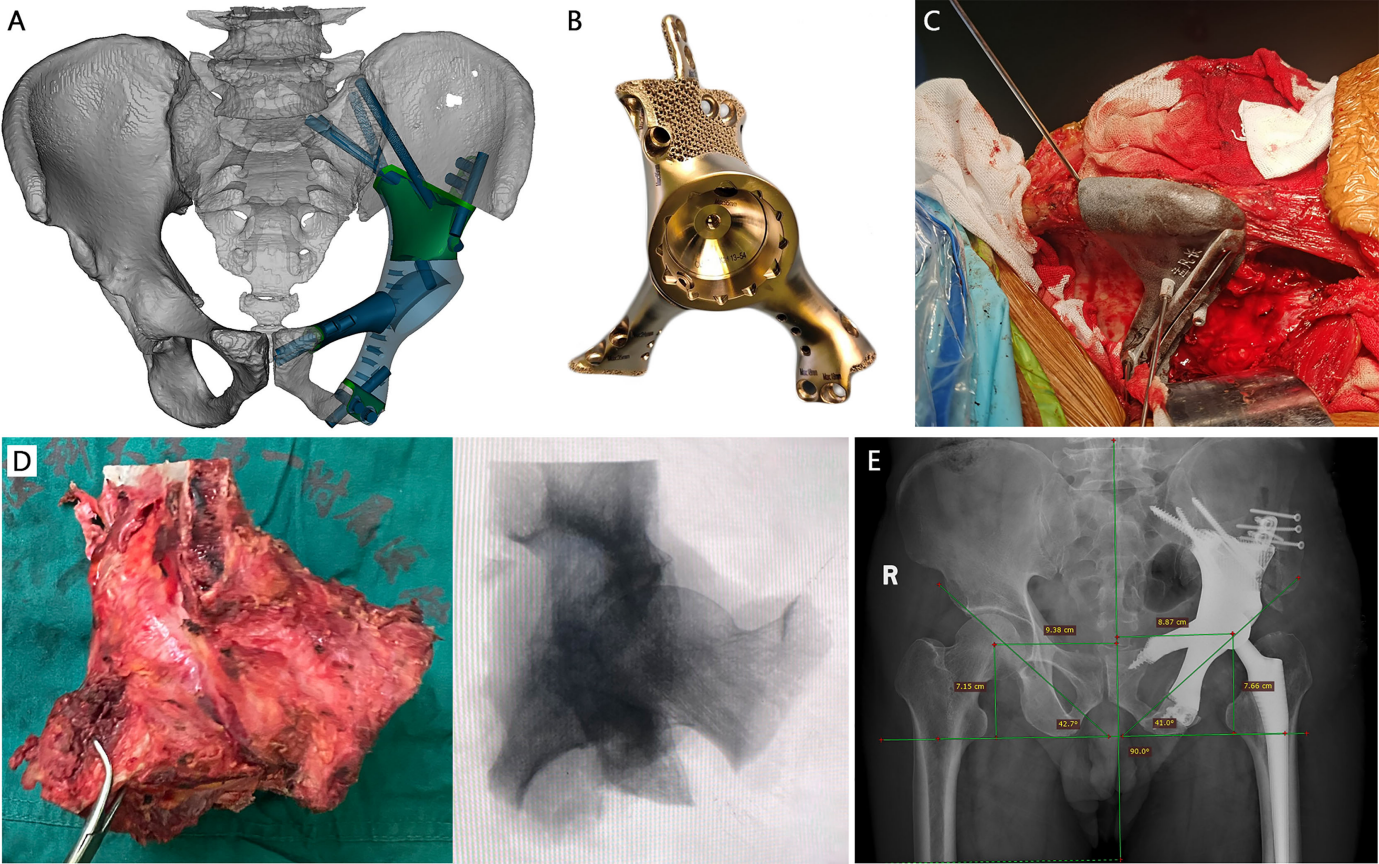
A 66-year-old male patient with metastatic prostate cancer. (A) The design of type B prosthesis after partial type I + II + III resection (green part: porous structure; blue part: solid structure). (B) The real picture of the implant. (C) Intraoperative installation of patient-specific osteotomy guide on the iliac crest. (D) Extra-articular resection of the tumor. (E) Postoperative x-ray manifested promising accuracy of the prosthesis.
